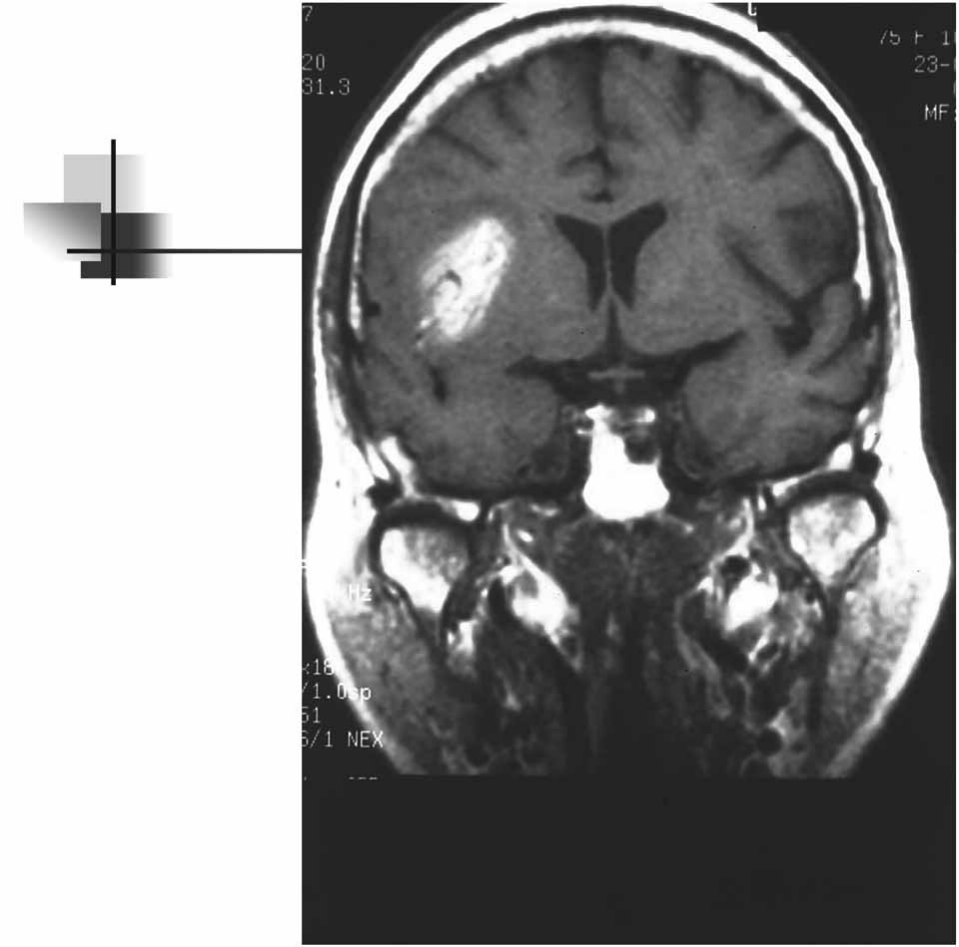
Hemorrhagic cardioembolic infarction in a patient with a spectacular shrinking deficit syndrome visualized in the brain MRI study (spin-echo hyperintensity T1-weighted image).
